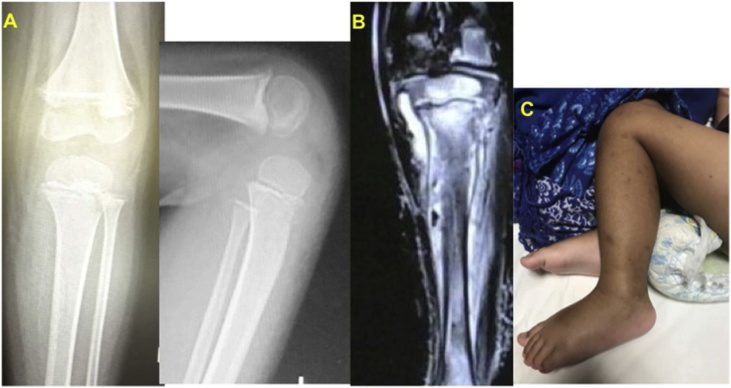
(A). Plain radiology findings: signs of osteopenia, the thick sclerotic metaphyseal line above a widened physis, and small beak-like excrescences at the metaphysis of both tibias, (B). High signal intensity and fluid-fluid levels on T2-weighted images on the proximal tibia. Based on MRI examination we suggest an osteomyelitis, (C). Clinical sign: swelling, unwilling to bear weight.
